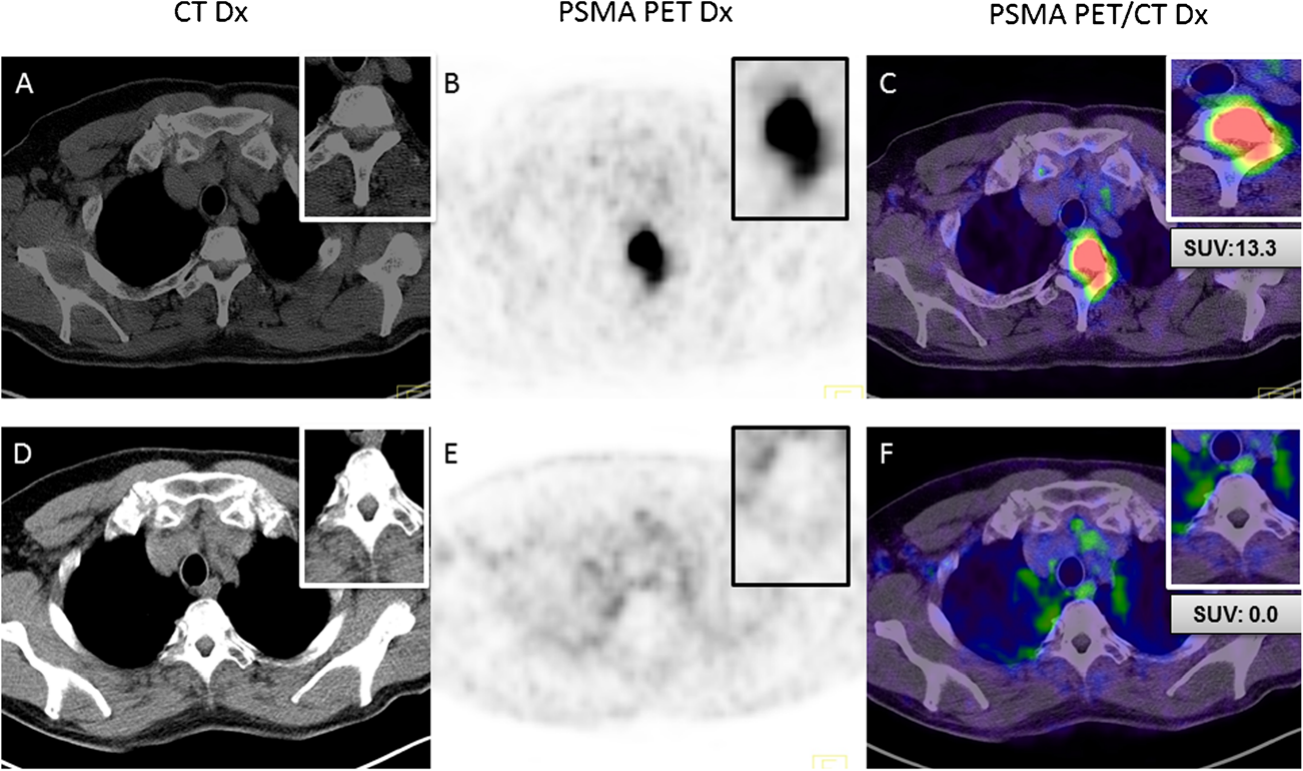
64-year old prostate cancer patient with a bone metastasis in T2, before PSMA-guided radiotherapy (a–c; SUVmax 13.3) and after irradiation (d–f; SUVmax 0)
